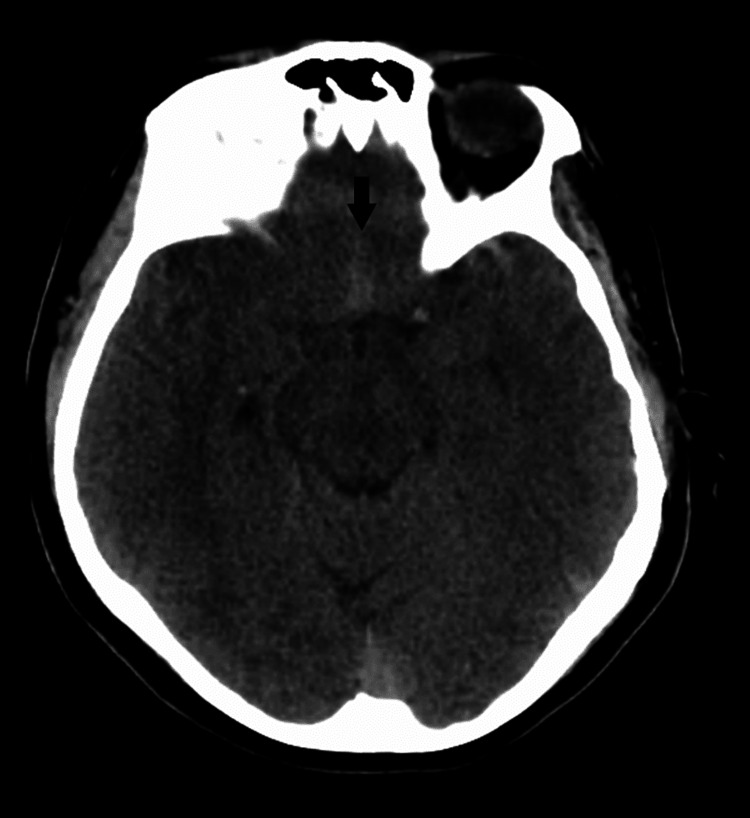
Pre-operative non-contrast CT head with identified subarachnoid hemorrhage in the interhemispheric fissure in the region of the anterior communicating artery. A pre-operative non-contrast CT head was obtained. An arrow points towards hemorrhage in the subarachnoid spaces and interhemispheric fissure in the region of the anterior communicating artery. CT: computed tomography
